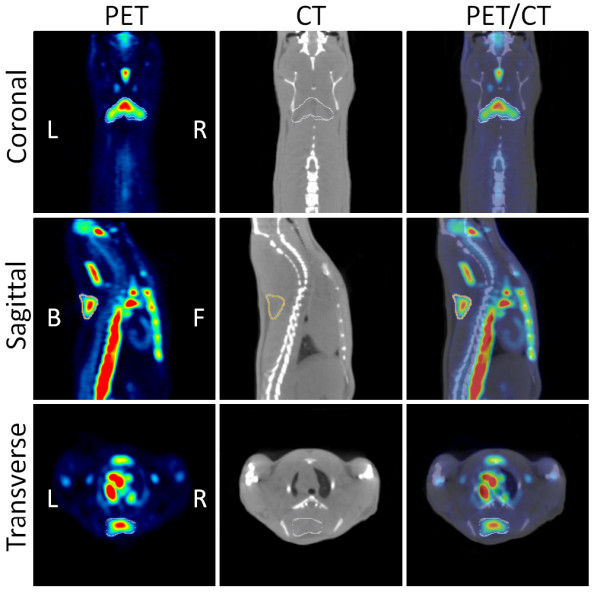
Coronal, sagittal, and transverse views of PET/CT images. The images show how automatic contour VOIs were drawn on the CL316,243-activated BATs: PET (left), CT (middle), and fused PET/CT (right).
